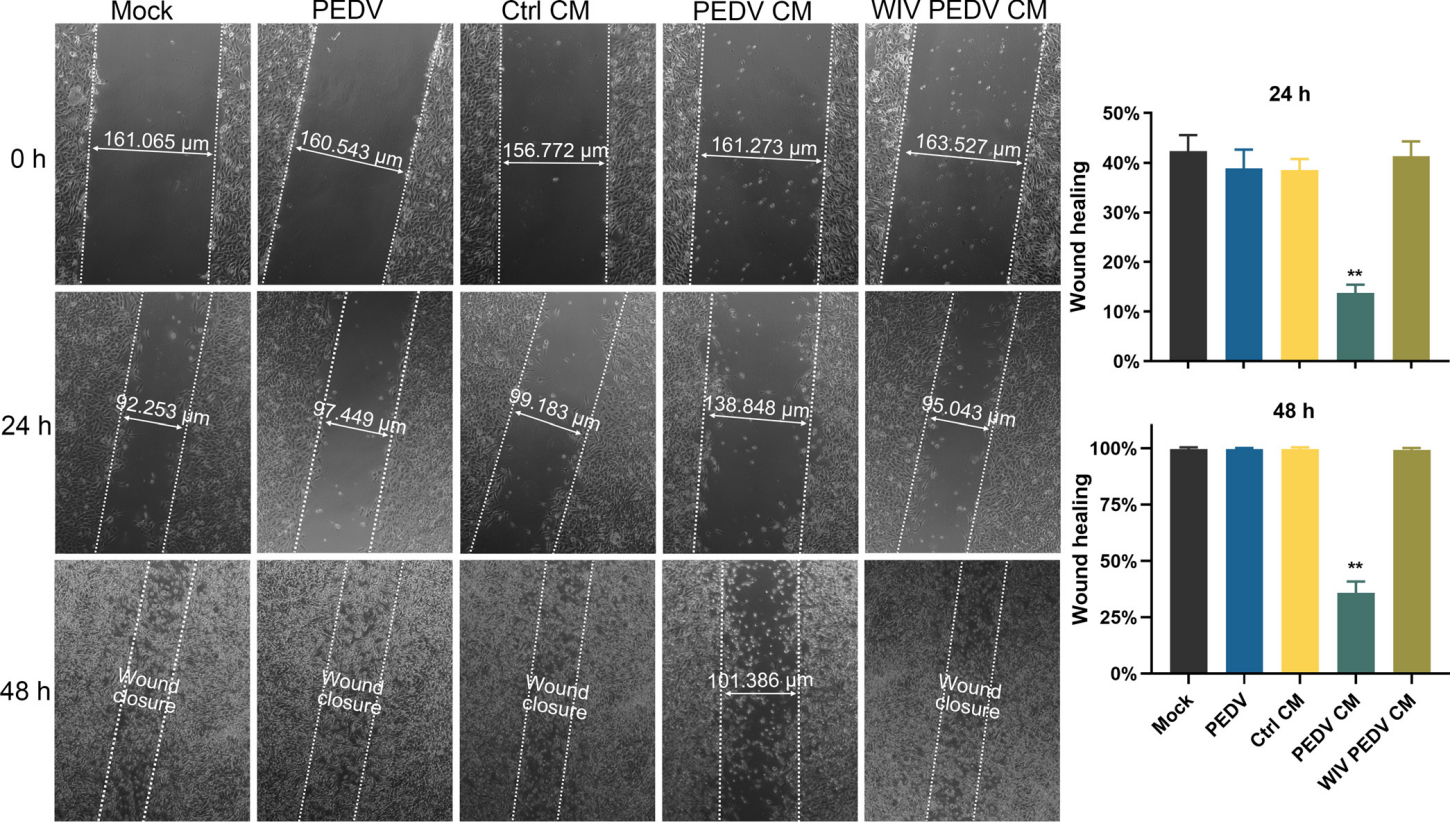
Molecules released by PEDV-infected NECs impede the endothelial barrier repair. After scratching, confluent SUVECs were inoculated with PEDV and incubated with mock, PEDV, or WIV PEDV CM. The wounds were measured using ZEN software. Quantification of wound recovery at 24 hpi and 48 hpi. Data are means and SD from three independent experiments. , *P < *0.01.
